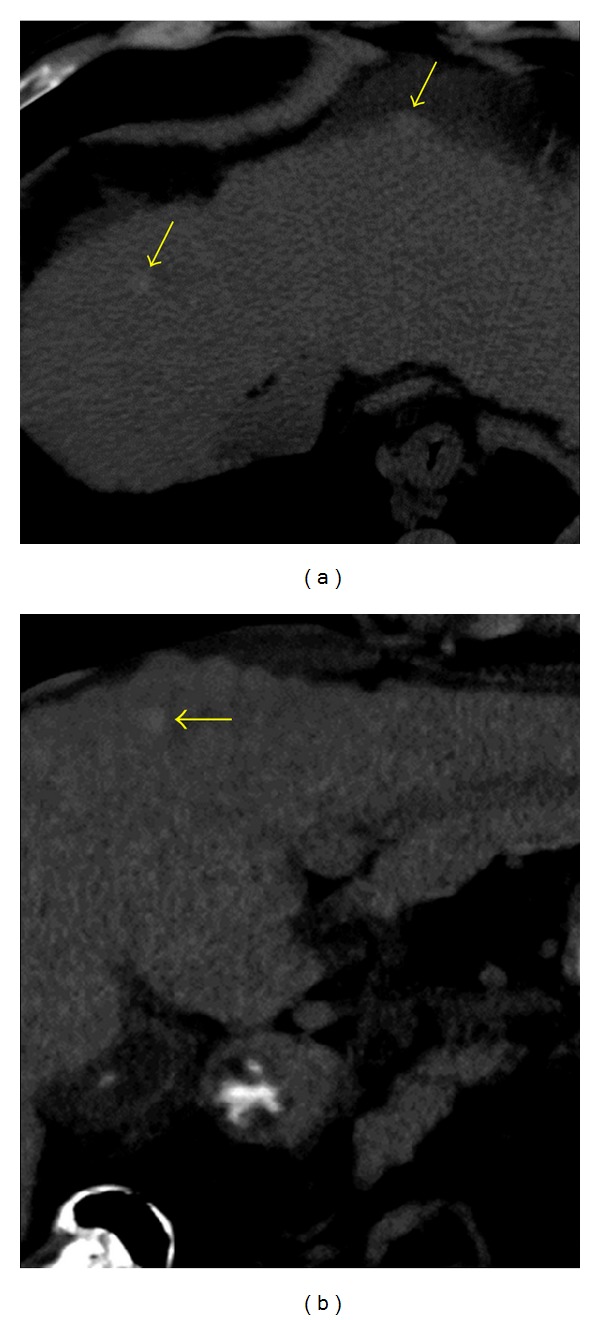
Siderotic regenerative nodules in a patient with cirrhosis. Unenhanced axial (a) and coronal (b) CT images show multiple subcentimeter high attenuation hepatic nodules (arrows). Note the nodular hepatic surface in this patient with micronodular cirrhosis.
